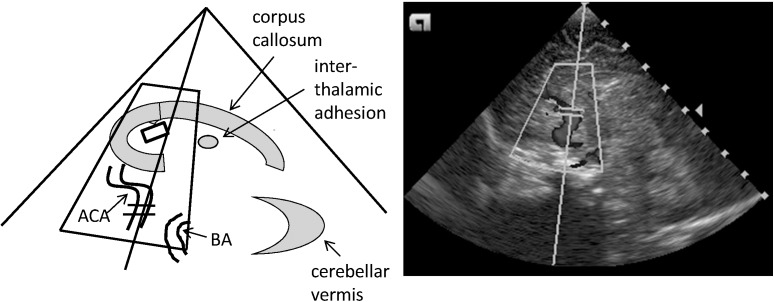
Standard sagittal plane of the cerebral color Doppler ultrasound investigation performed through the anterior fontanel. This midline sagittal image shows the corpus callosum, the interthalamic adhesion, and the cerebellar vermis as reference structures. ACA, anterior cerebral artery; BA, basilar artery.
